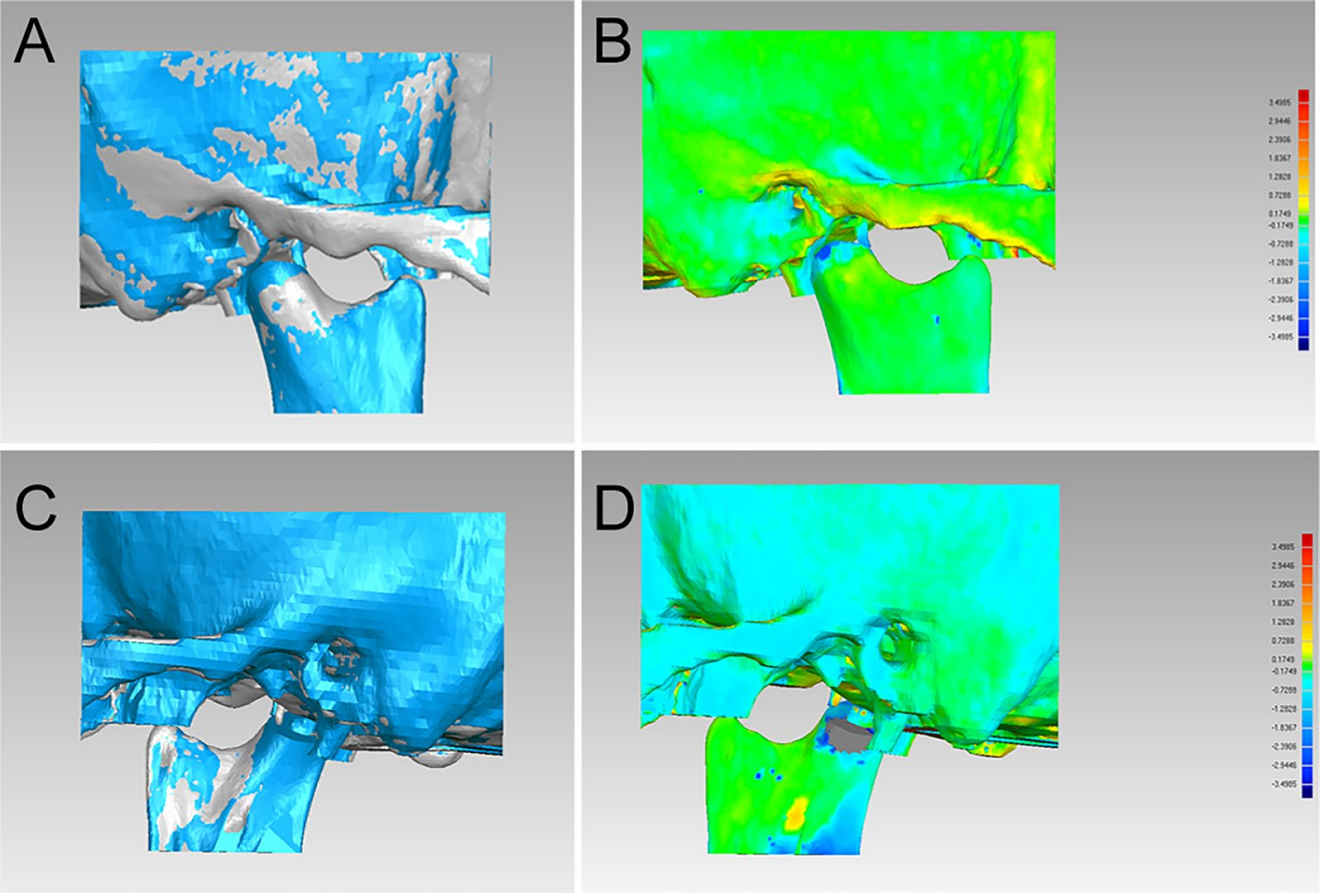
Evaluation of the accuracy of condylar repositioning guide. a, c superimposition of the virtual plan (blue) and postoperative CT scan at 3 days after surgery (grey); b, d the postoperative accuracy study of condylar repositioning guide using the Geomagic Studio 2013 software. e, f the magnification view of part of the zygomatic arch, fossa, and neocondyle
